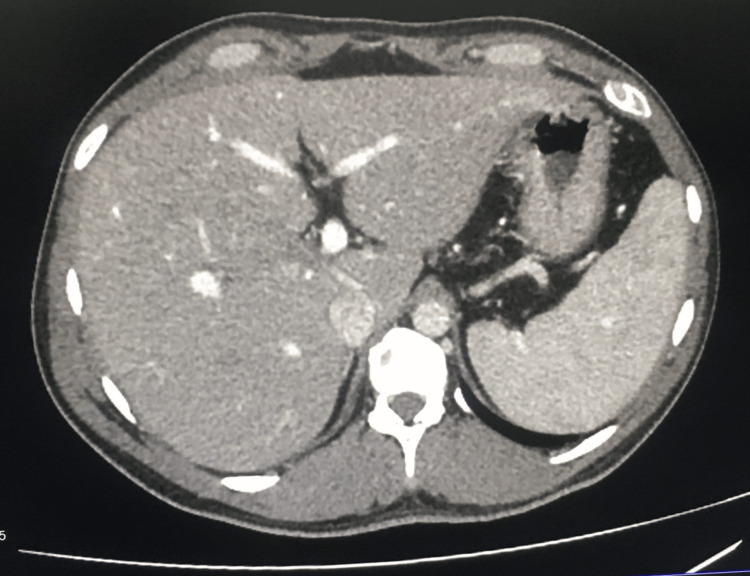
Contrast CT abdomen with mild hepatomegaly and early fatty liver changes
